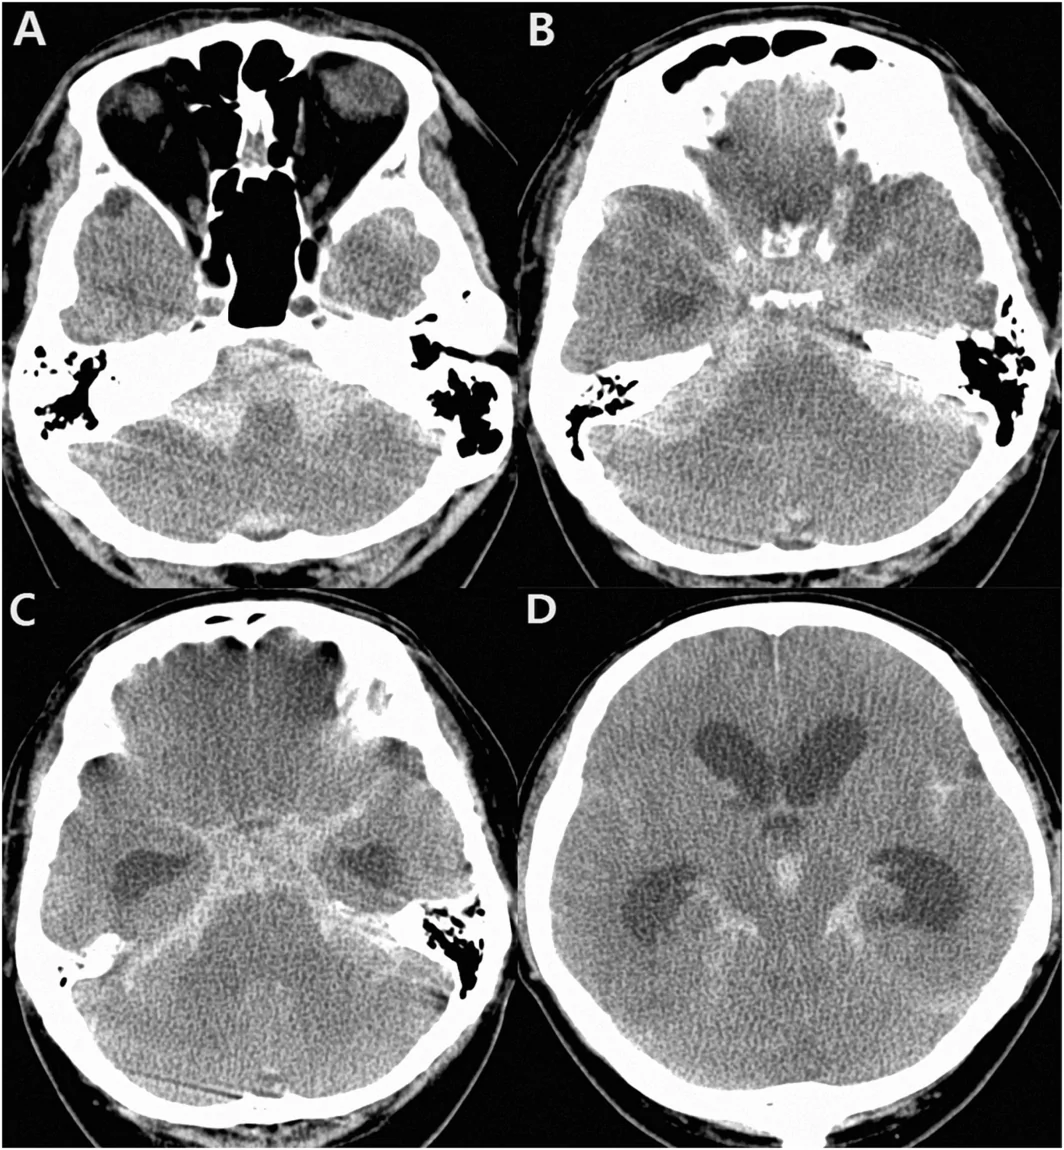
Axial brain computed tomography images obtained after neurological deterioration, with subarachnoid hemorrhage detected after percutaneous coronary intervention, showing diffuse thick subarachnoid hemorrhage centered in the basal cisterns, bilateral Sylvian cisterns, and anterior brainstem cisterns, with intraventricular extension and acute hydrocephalus, consistent with modified Fisher grade 4 subarachnoid hemorrhage (A–D).
